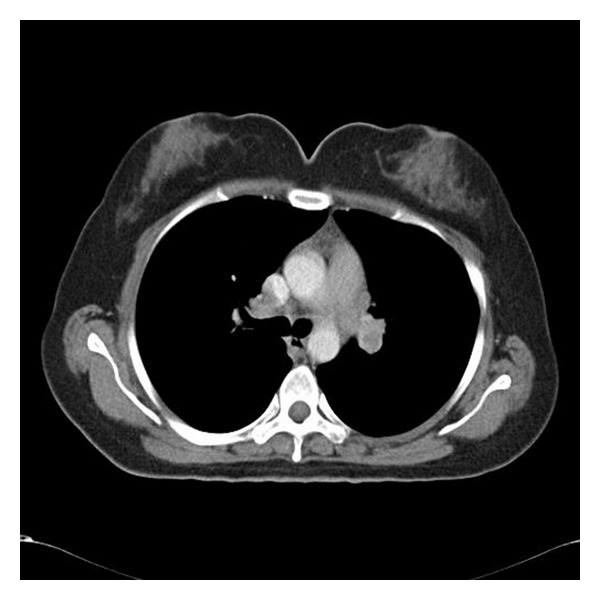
Bilateral hilar lymphadenopathy on thoracic CT.
